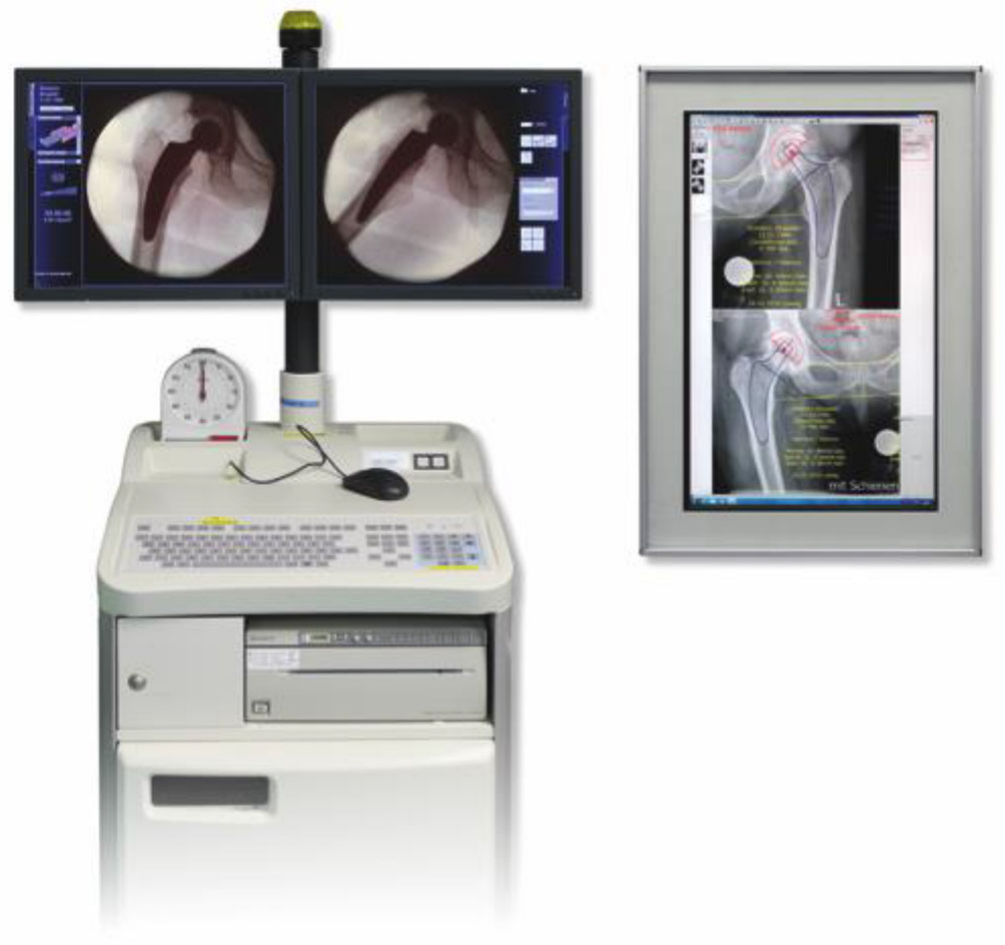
After inserting a head and performing a trial reduction, the assessment of an intraoperative single shot radiograph using a digital image intensifier is highly recommended at this point in order to compare the positioning of the rasp (trial implant) to the preoperative templating. Offset and leg length can be verified and, if necessary, changes can be made. Note that risk of postoperative subsidence might be increased in cases not reaching cortical contact. This should be taken into consideration choosing the correct implant size. (Courtesy of Mathys Ltd Bettlach)
